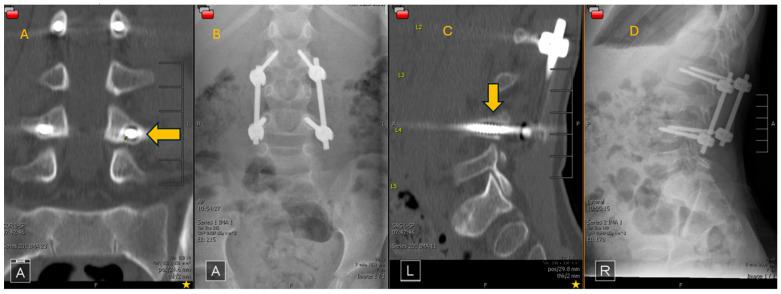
Post-operative CT and upright X-ray lumbar films. (A) AP film showing haloing around the left L4 screw, (B) AP representative X-ray, (C) Sagittal film focused on left L4 screw with arrow showing haloing, (D) Sagittal representative X-ray.
